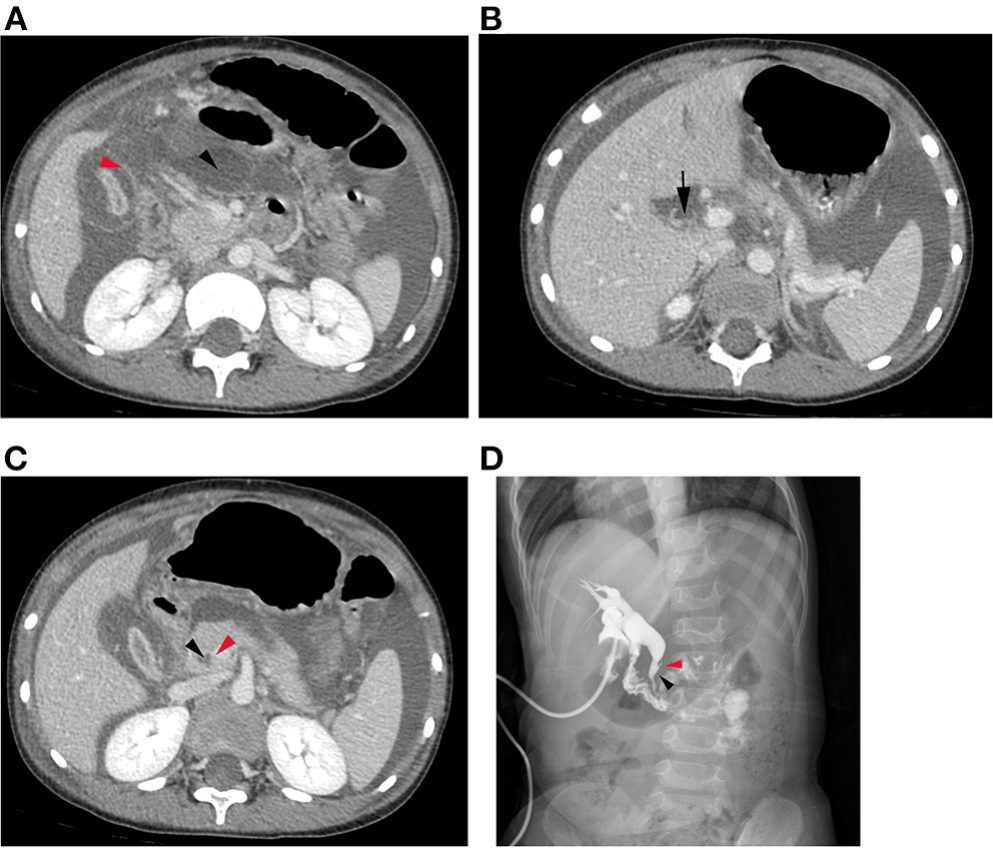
(A) Abdominal CT examination before surgery revealed thickened gallbladder wall with edema, narrow gallbladder cavity, and reinforced gallbladder mucosa (red arrow). Hydrops were found in the abdominal cavity and lesser omental bursa (black arrow). (B) A common bile duct rupture was observed in large perforations (black arrow). (C) Abdominal CT examination before surgery revealed that the pancreatic duct (red arrow) merged with the common bile duct (black arrow) in advance in cases with abnormal bile pancreatic confluence. (D) Cholangiography revealed that the pancreatic duct merged with the common bile duct in advance in cases with PBM.
